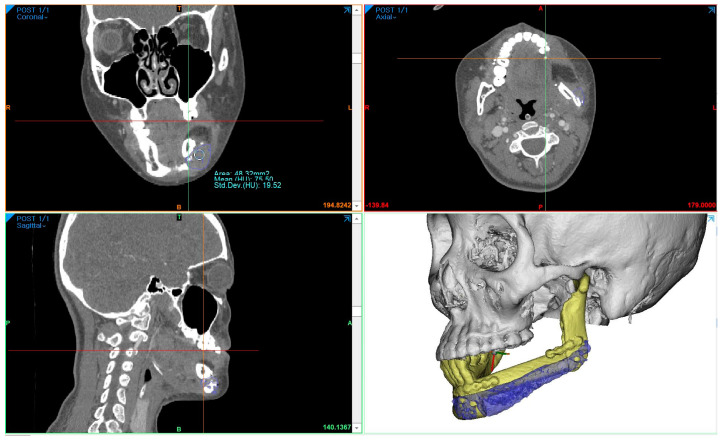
Using the “density” function in Mimics software, the Hounsfield units’ value of the PCL implant area was obtained at the same location. In this case, the measurement of the same region was conducted at the level determined by the location of the hemoclip below the fibula segment and the maxillary first molar in the CT coronal view.
